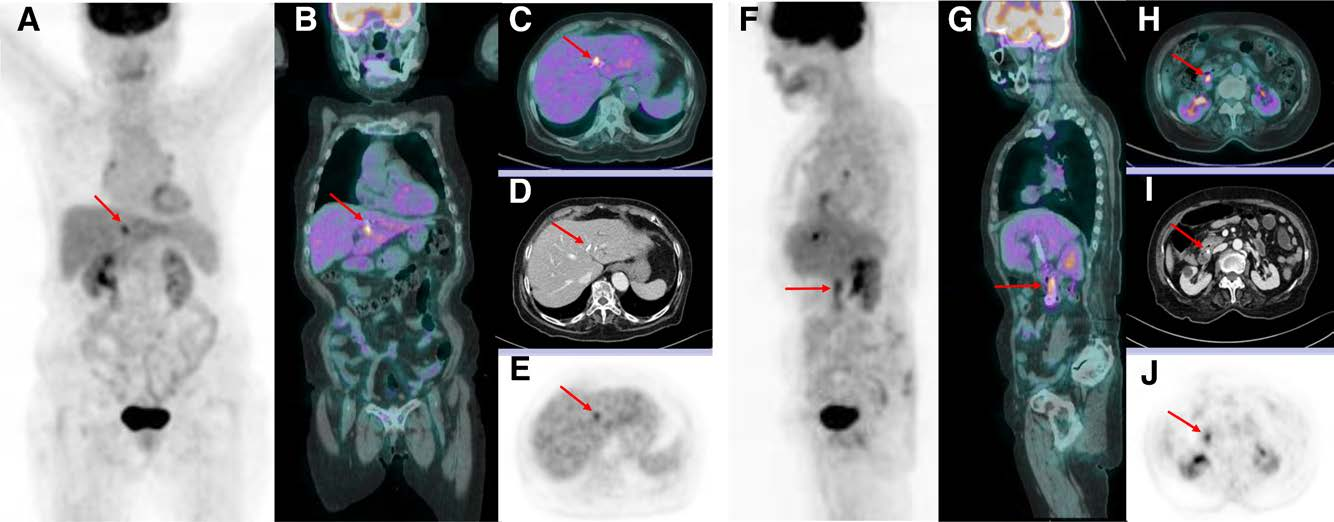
Given her persistent fever of unknown origin, FDG PET/CT was performed to identify occult infection. The scan demonstrated focal FDG uptake at the proximal tip of the stent in the left intrahepatic bile duct (A, coronal maximum intensity projection, B, coronal fusion, C, axial fusion, D, axial CT, and E, axial PET images, red arrows) and linear hypermetabolism along the stents in the distal CBD (F, sagittal maximum intensity projection, G, sagittal fusion, H, axial fusion, I, axial CT, and J, axial PET images, red arrows), suggestive of infected biliary stents. CBD = common bile duct, CT = computed tomography, FDG = fluorodeoxyglucose, PET = positron emission tomography.
